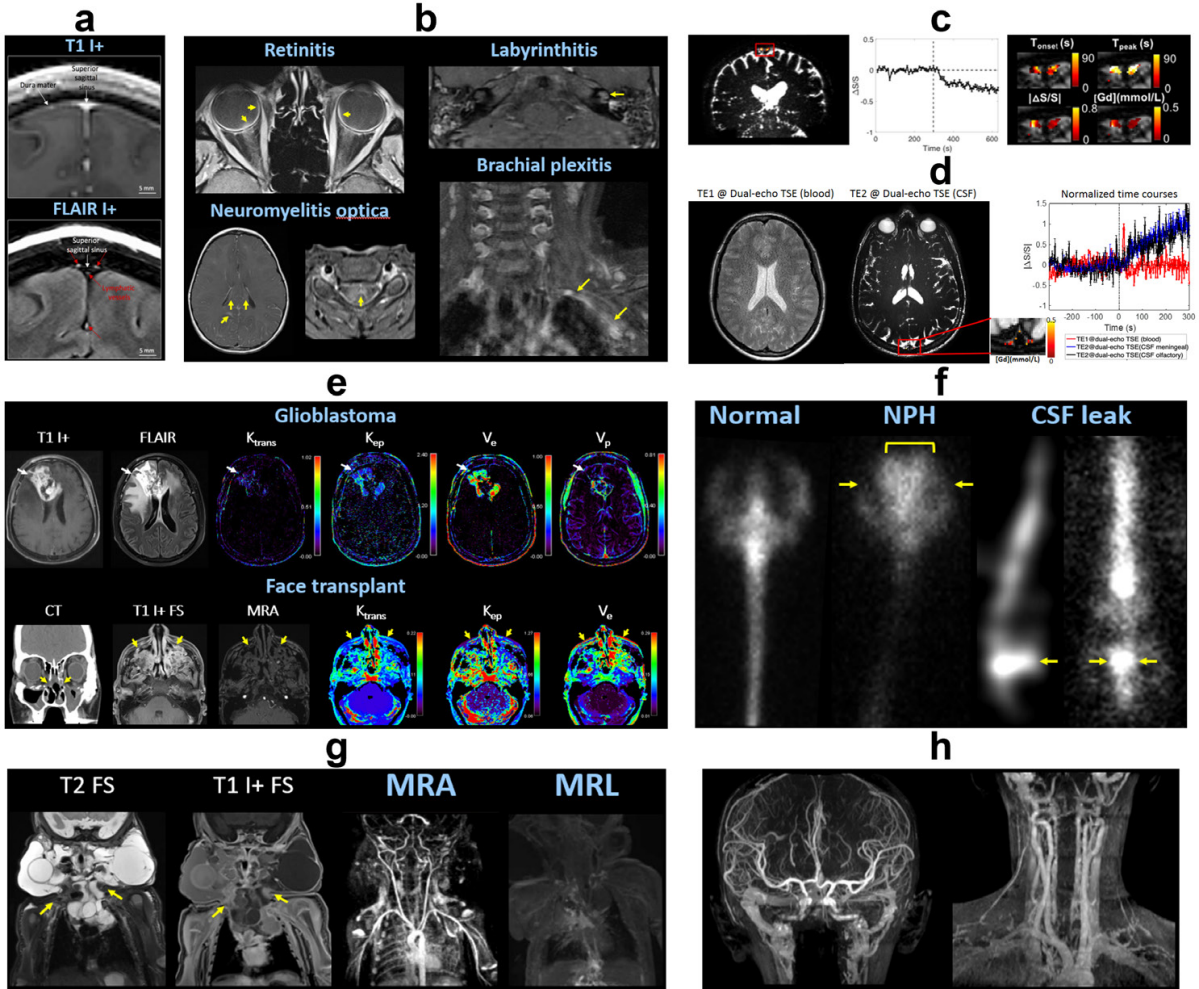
Contrast-enhanced imaging. (a) Meningeal lymphatics. Post-contrast T1-weighted MRI (top) shows enhancement of the dura mater and vascular structures (arrows). Post-contrast FLAIR (bottom) highlights the meningeal lymphatics (red arrows) in the parasagittal dural space, located between the cortical venous walls (white arrows) and pia mater. (b) Post-contrast T1-weighted MRI showing blood–neural barrier breakdown with abnormal enhancement in COVID-19 retinitis, left labyrinthitis, neuromyelitis optica, and left brachial plexitis (arrows). (c) DSC MRI using T2-weighted TSE shows dynamic signal changes in meningeal lymphatics (red box) surrounding the superior sagittal sinus. By plotting the signal over time relative to Gad injection (dotted line), several parameters can be extracted including T_onset_ = onset time, T_peak_ = time to peak, absolute value of relative signal change |ΔS/S|, and [Gd] = concentration of Gad. (d) Dual-echo TSE MRI can measure Gad-induced signal changes in blood at short TE (TE1) and CSF at longer TE (TE2), providing information about dynamic flow through blood vessels and meningeal lymphatics in a single scan. (e) DCE MRI of glioblastoma and facial transplant shows alterations (arrows) of various parameters including plasma volume V_p_, interstitial volume V_e_, transfer constants K_trans_ (from plasma to ISF) and K_ep_ (from ISF to plasma). (f) Radionuclide cisternography. Left panel: Healthy subject shows ascent of injected radiotracer through the lumbar spine to basal cisterns, cerebral convexities, and vertex. Central panel: Normal pressure hydrocephalus shows abnormal reflux into the lateral ventricles (bracket) and impaired ascent over the cerebral convexities (arrows). Right panel: Lumbar CSF leak with contrast pooling (arrows) and delayed ascent. (g) MRI, time-resolved MRA, and MRL of lymphatic malformation involving the neck and upper chest (arrows). There is Gad leakage within the malformation and disorganized drainage to the peripheral lymphatic system. (h) Ferumoxytol MRA. Arterial, capillary, and venous systems are concurrently opacified due to the long blood pool residence time. DCE, dynamic contrast-enhanced; DSC, dynamic susceptibility contrast; FLAIR, fluid-attenuated inversion recovery; MRL, MR lymphangiography; TE, echo time; TSE, turbo spin echo.
